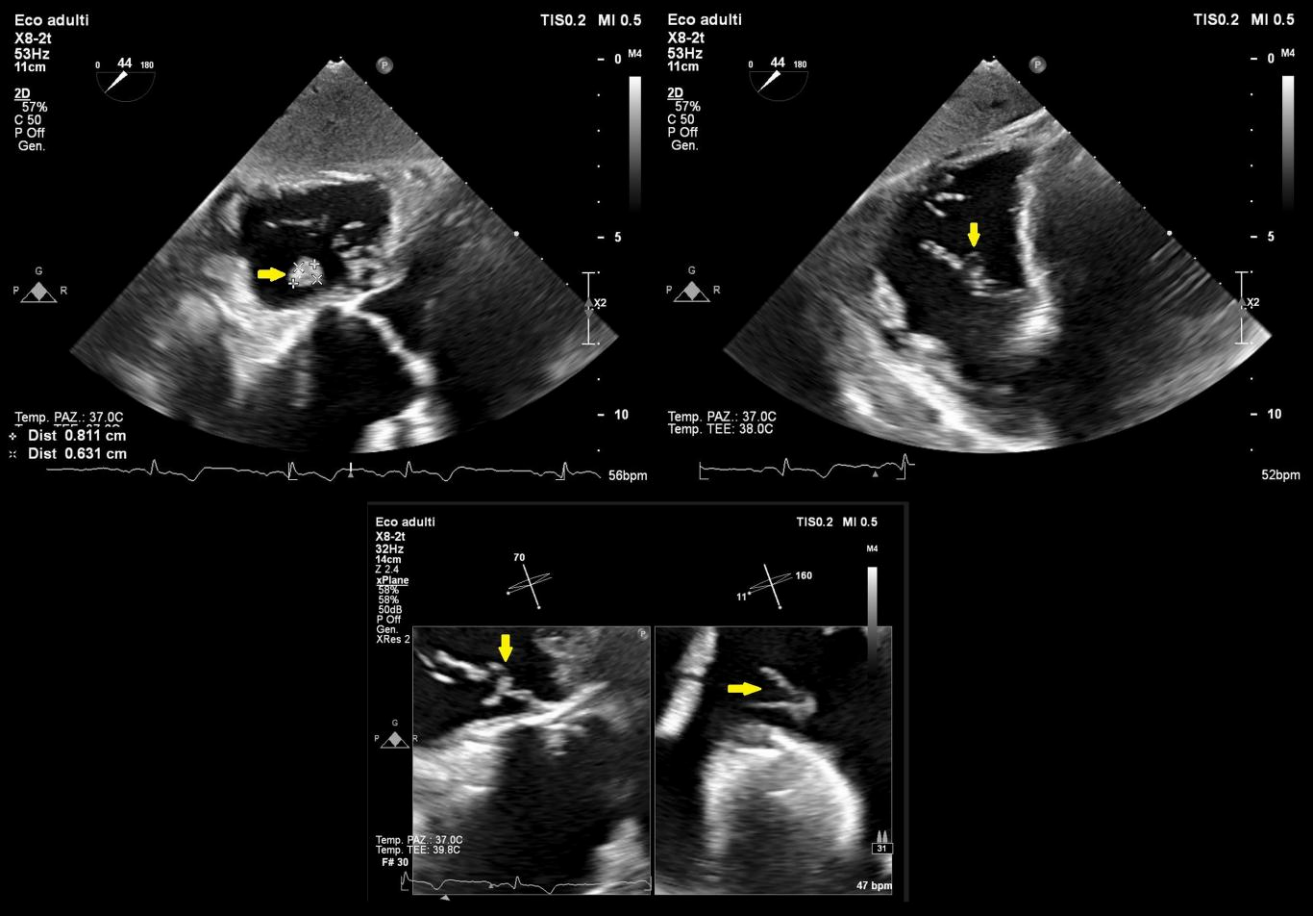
Different short axis views of the tricuspid valve from the transoesophageal transgastric window, showing the tricuspid valve aneurysm.
